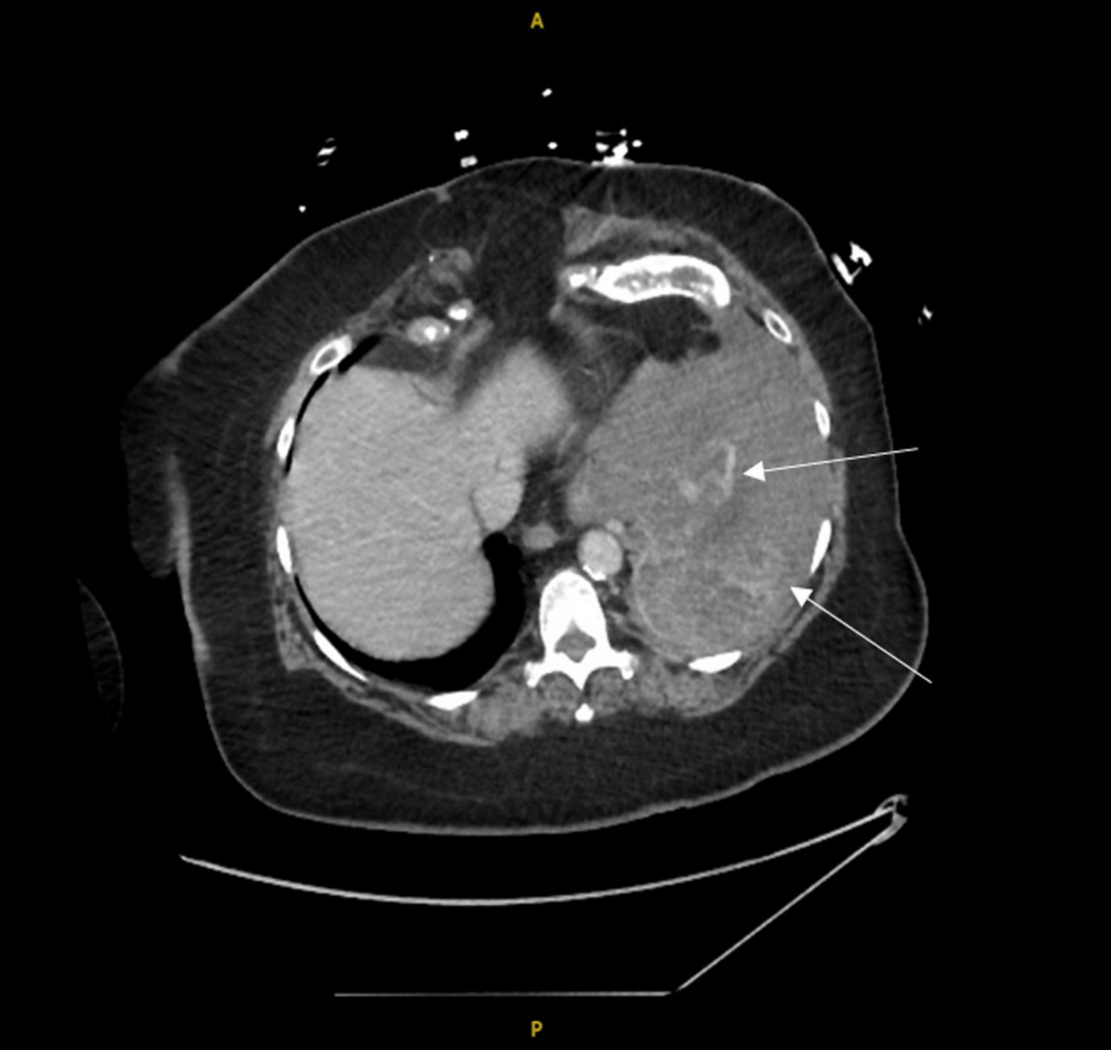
Computed tomography angiography axial image. Delayed sequence imaging demonstrating swirling contrast within the hemothorax, consistent with active hemorrhage (arrows).
